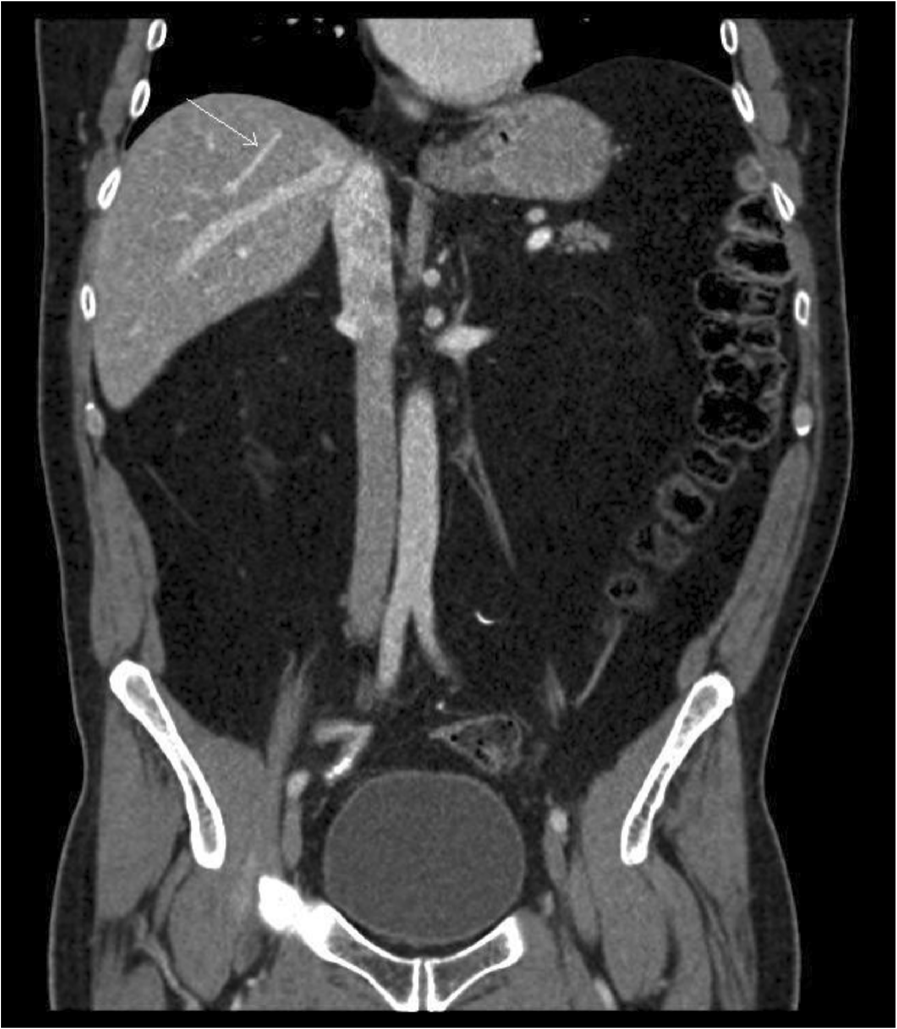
CT scan showing large middle vein tributary (arrow) draining the right liver.
